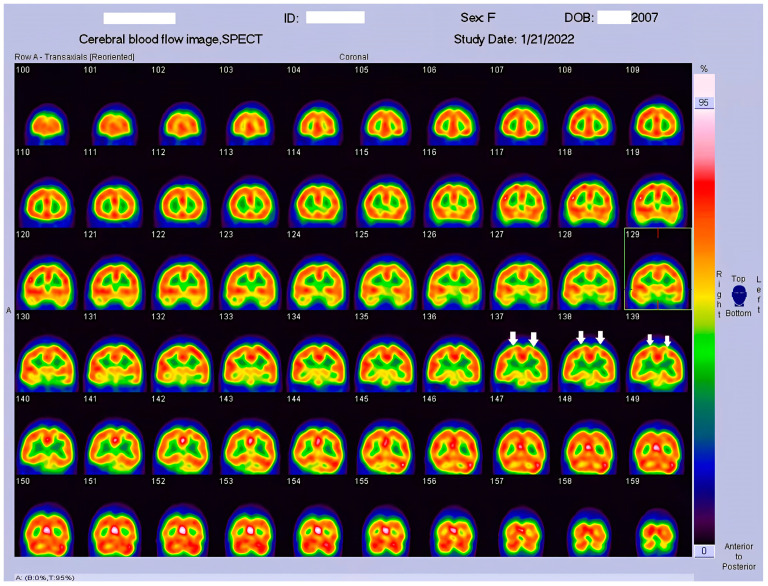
Coronal view of the Tc−99m ECD brain perfusion SPECT images. A decreased uptake in the tracer can be observed in the parietal area, especially in the postcentral gyrus (arrow).
